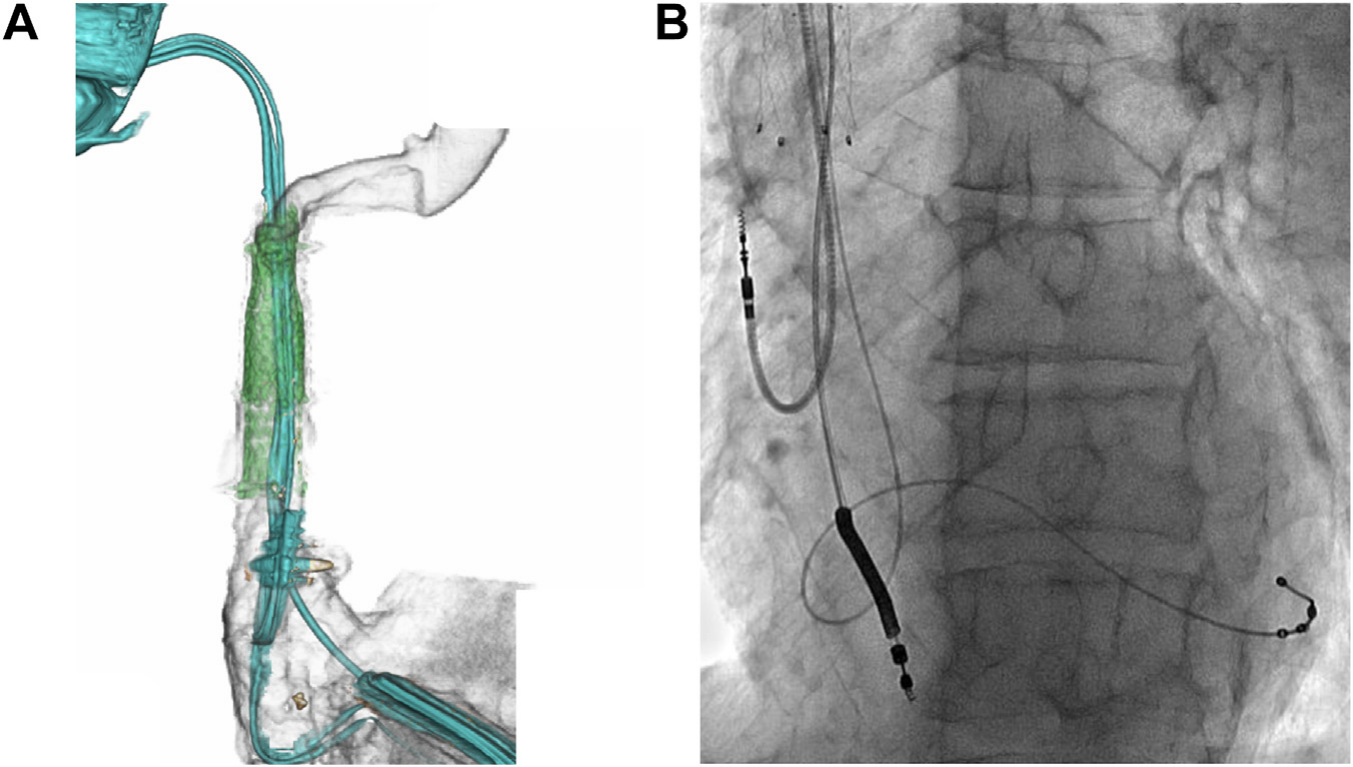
Postprocedural images. A: Computed tomography showing improved expansion of the right brachiocephalic vein and superior vena cava with stents. B: Fluoroscopic image showing a new cardiac resynchronization therapy-defibrillator system, with leads advanced through the newly stented veins (left anterior oblique view).
